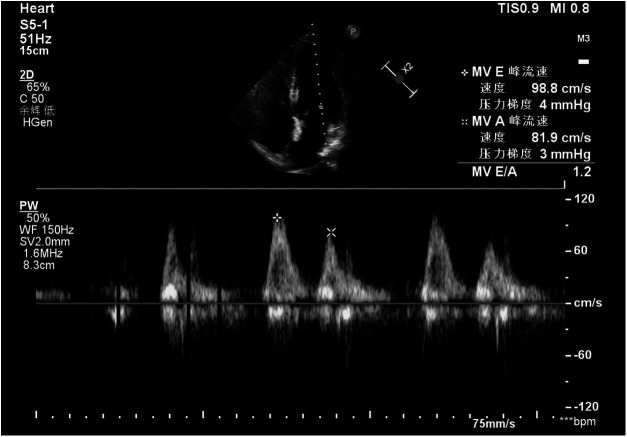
The echocardiographic image of the patient reveals a linear mass within the right atrium.
